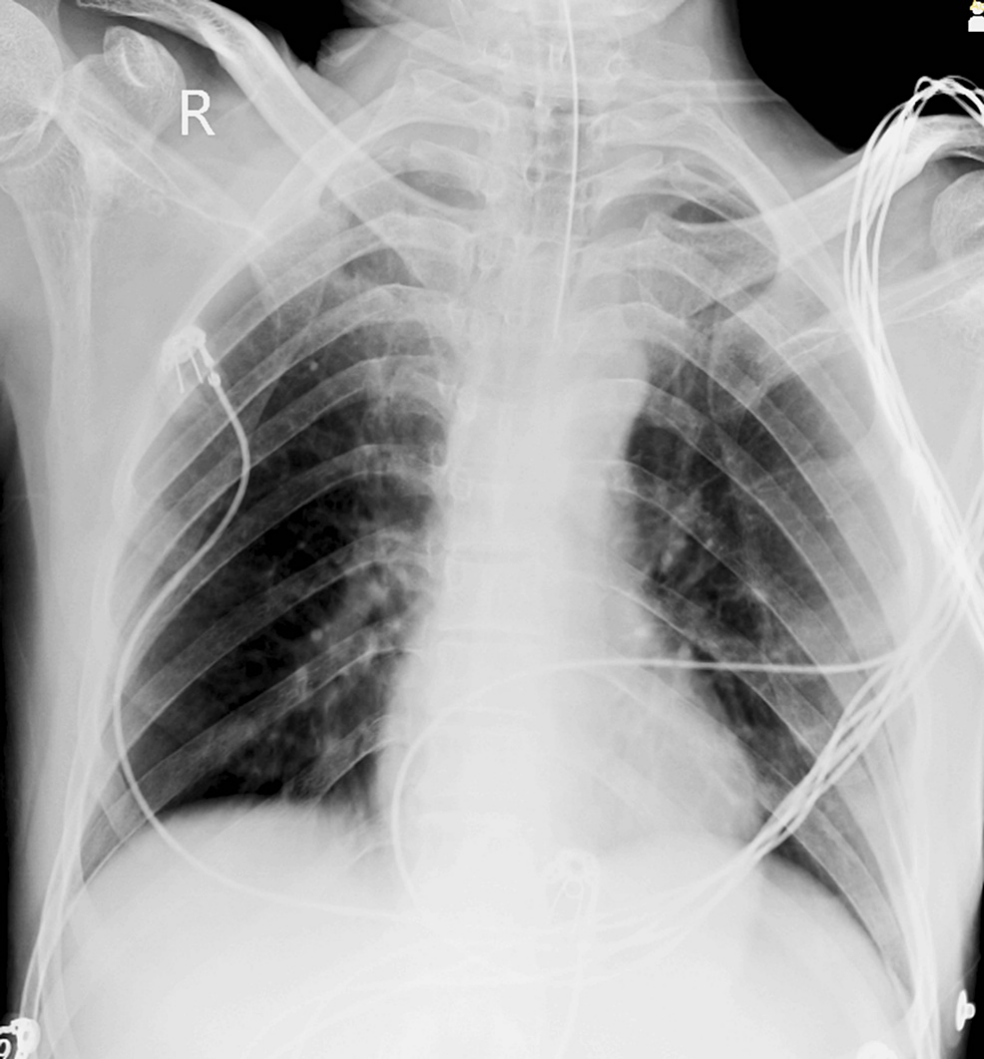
The chest X-ray of the patient showed normal findings.
